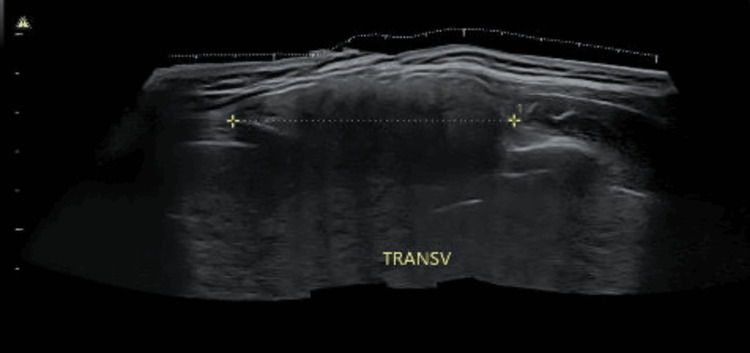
Ultrasound image of the elastofibroma This study revealed a lesion deep to the muscular planes, characterized by an irregular morphology, partially defined margins, and alternating hyperechoic and hypoechoic linear tracts without Doppler vascularity, measuring approximately 5.2 × 7.5 cm. These findings were highly suggestive of elastofibroma dorsi versus sarcoma.
